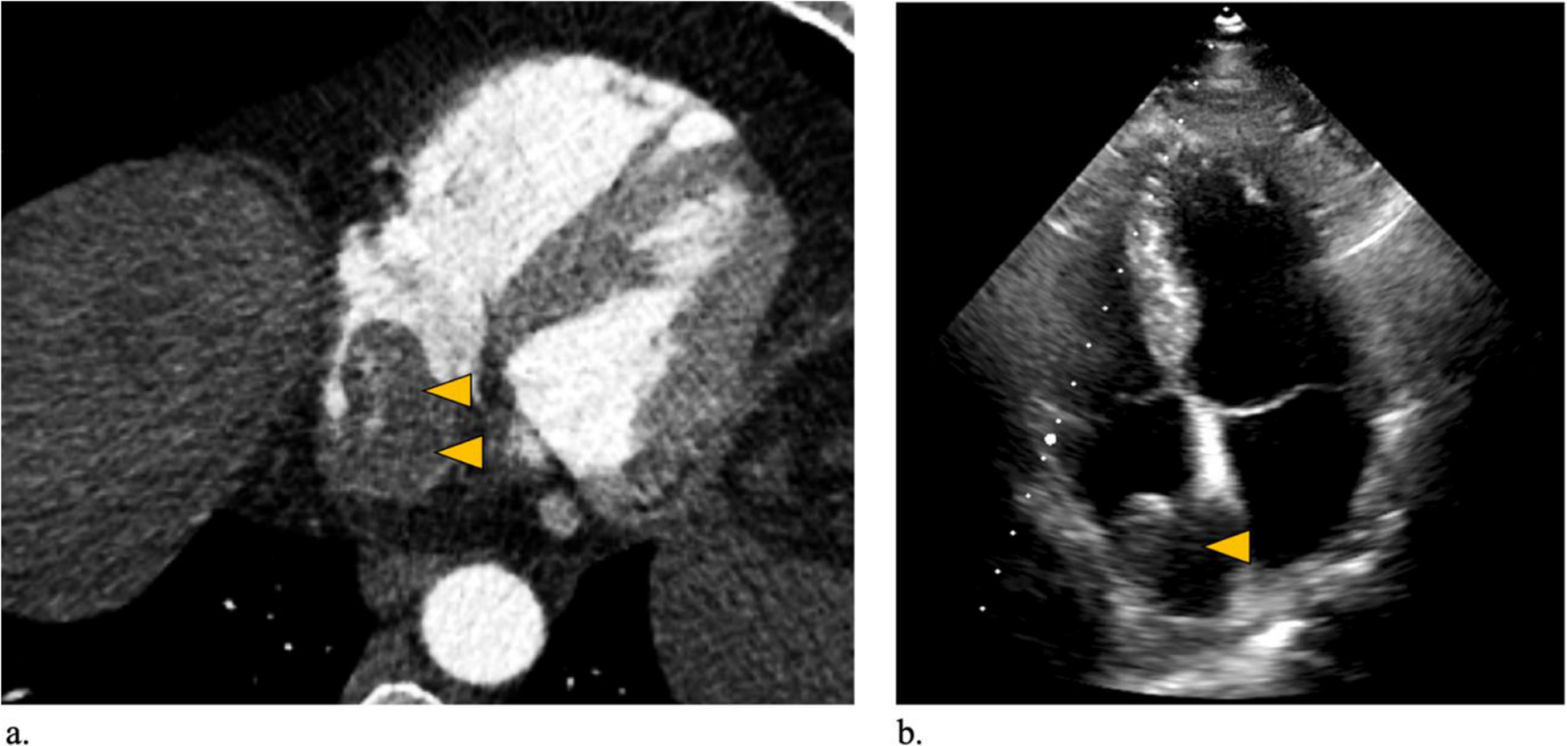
Pre-interventional imaging of the TT in the RA. (A) Pre-interventional axial CT-angiography showing extensive growth of the TT in the RA and confirming arterial enhancement in the TT. (B) Pre-interventional echocardiogram confirming a mass in the RA
